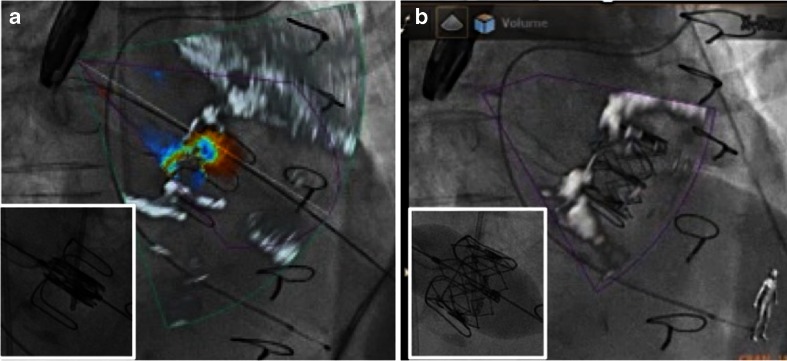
Fusion imaging before (a) and after (b) transapical mitral valve-in-valve replacement. a Fusion of 3D color Doppler transesophageal echocardiography with fluoroscopy: a failing 27-mm stented Edwards bioprosthesis in mitral position with flail of the lateral leaflet, leading to severe transvalvular mitral regurgitation. b After transapical valve-in-valve replacement, fusion imaging documents correct position of the implanted 26-mm Edwards Sapien XT bioprosthesis with complete leaflet coaptation and no residual regurgitation. *Small inlets* show fluoroscopy alone immediately before (a) and at the end (b) of the implantation of the Sapien XT prosthesis into the failing bioprosthesis
